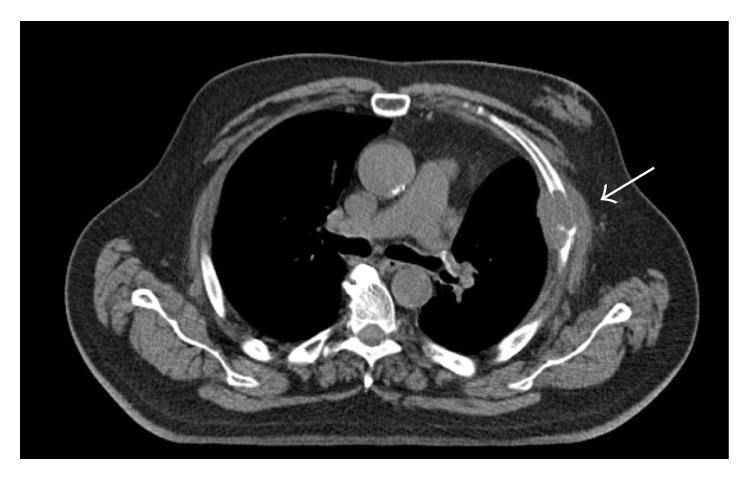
Computed tomography scan of the thorax showing left-sided local relapse in the pleura and thoracic wall after lobectomy. Status before palliative radiotherapy.
